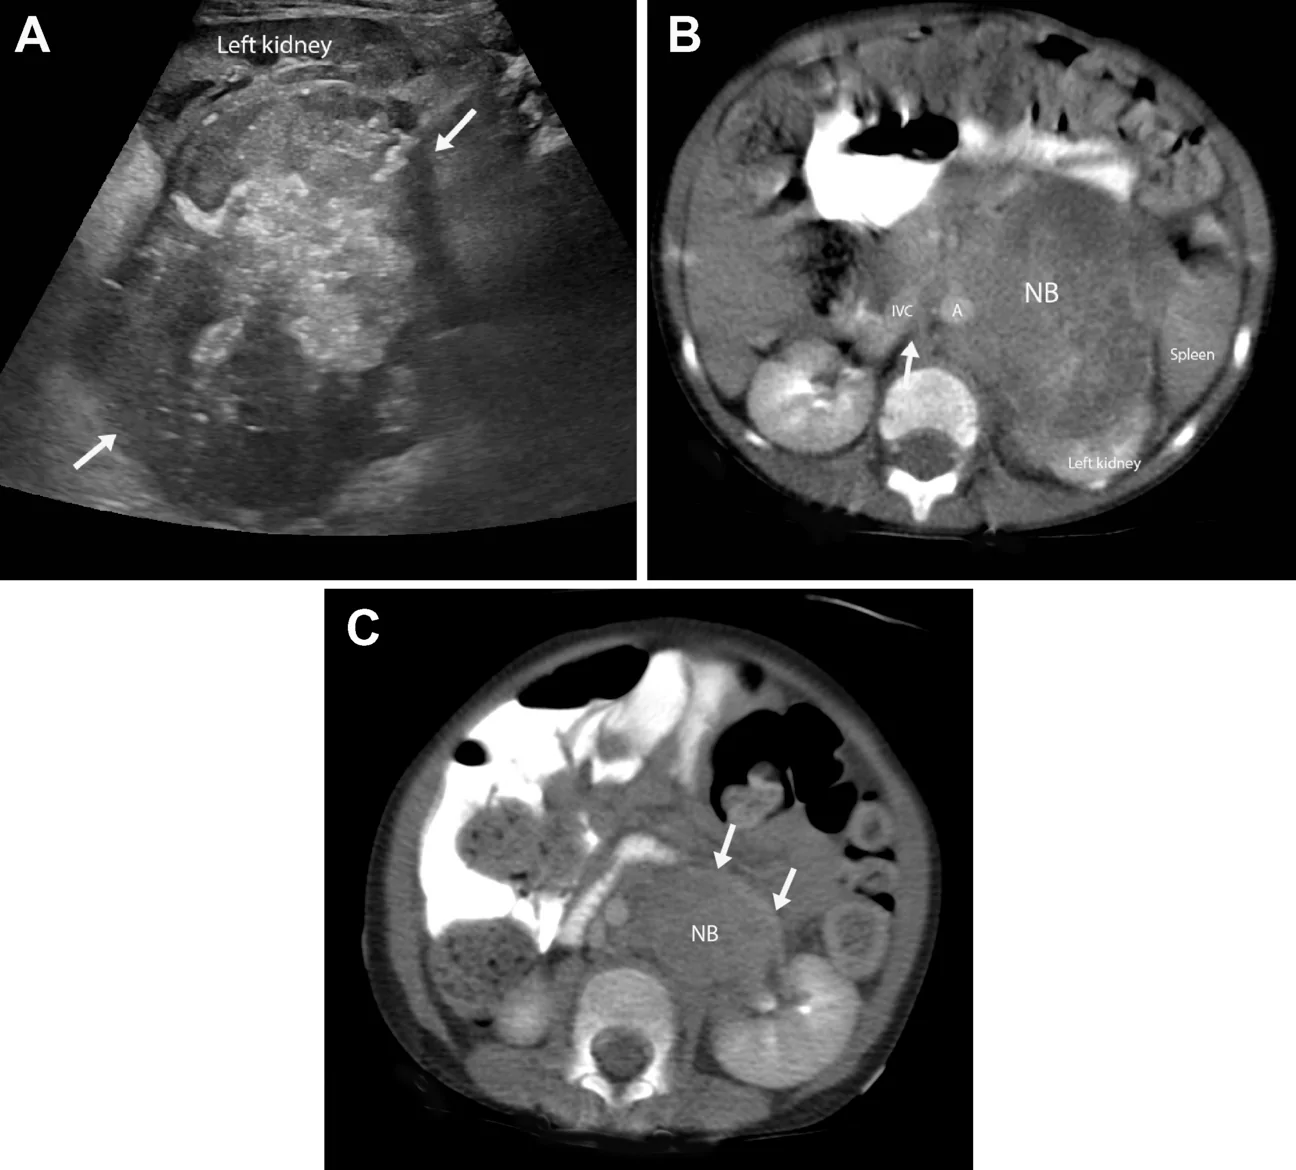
Neuroblastoma diagnosed in a 2-year-old male presenting with a palpable abdominal mass. a Abdominal ultrasound in longitudinal plane shows an ill-defined heterogeneous mass (*arrows*) in the left retroperitoneum displacing the left kidney anteriorly. Irregular echogenicities with posterior acoustic shadowing within the mass are consistent with calcifications. b, c Contrast-enhanced CT of the abdomen in axial plane at the (b) mid-level of the mass and (c) lower level of the mass shows heterogeneity with non-enhancing, low-attenuating regions consistent with cystic components, possibly necrosis. *NB*, neuroblastoma. Notable image-defined risk factors include neoplasm surrounding the abdominal aorta (*A*) and abutting at least 50% of the surface of the IVC (*arrow*, b). The left renal vein is displaced anteriorly and stretched over the ventral aspect of the mass (*arrows*, c)
